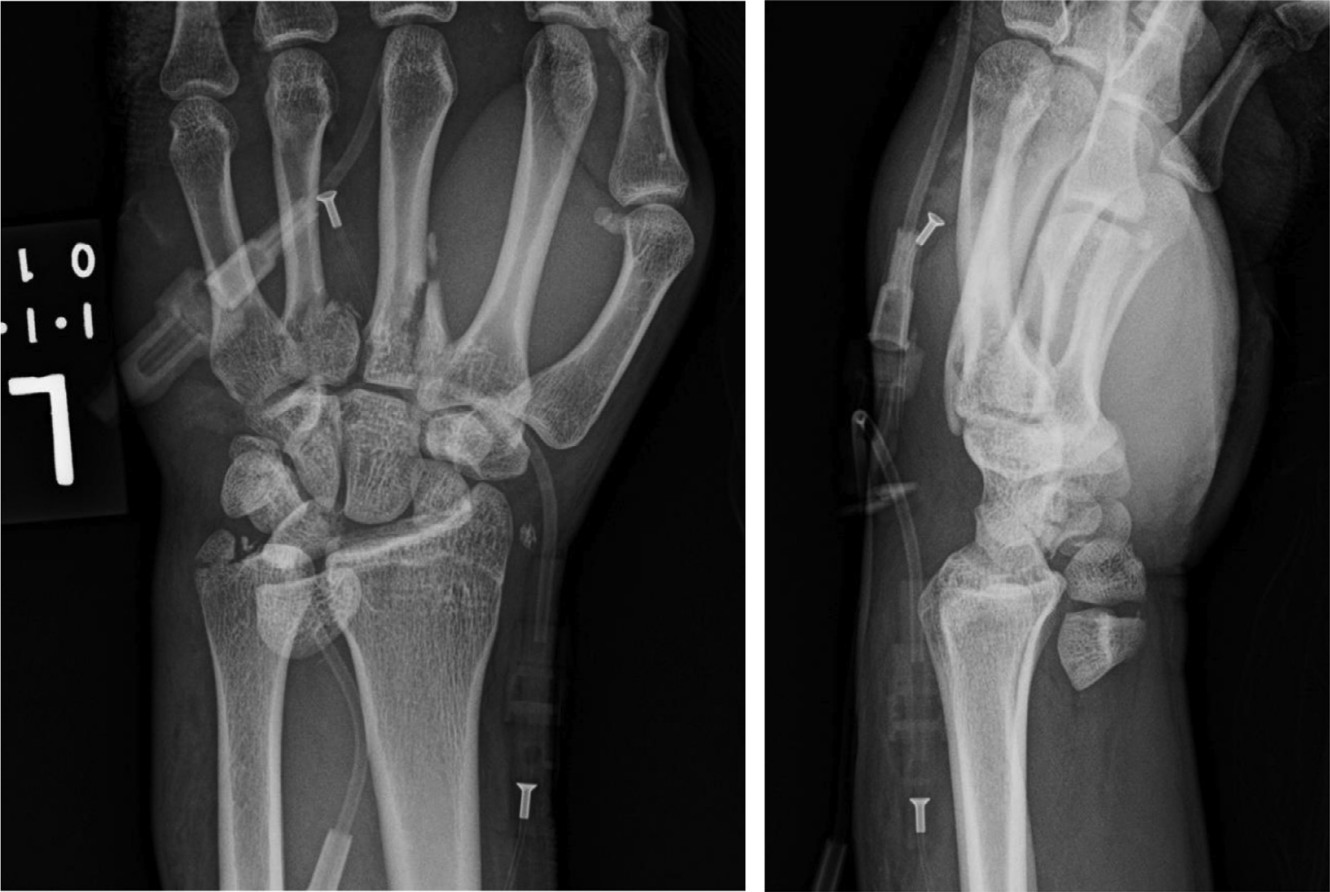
Patient 3: Initial radiographs of the left wrist demonstrating a trans-ulnar styloid trans-scaphoid lunate dislocation. A nondisplaced triquetral fracture was noted on subsequent CT. There are also fractures of the third and fourth metacarpal bases.
